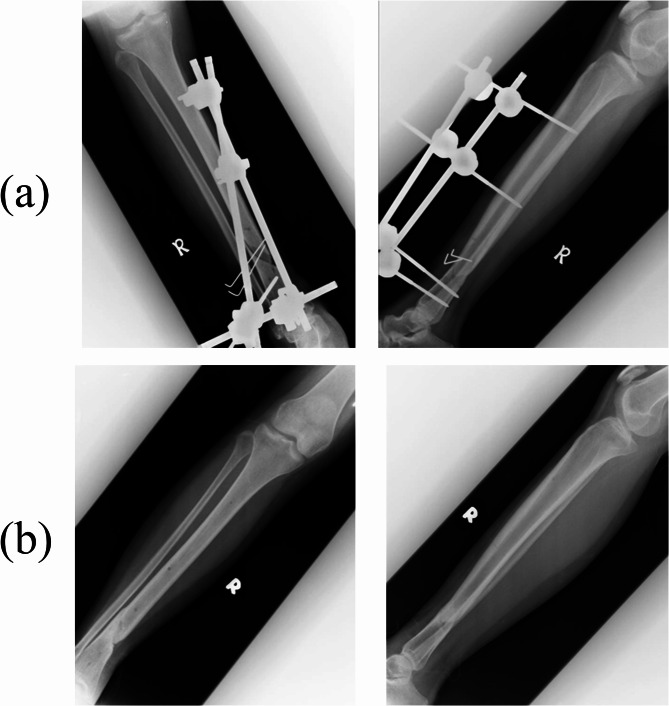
Radiographs of the single crossed locked rod in a comminuted distal 1/3 tibia fracture done on Patient B; (a) post-operative and (b) after heal.
